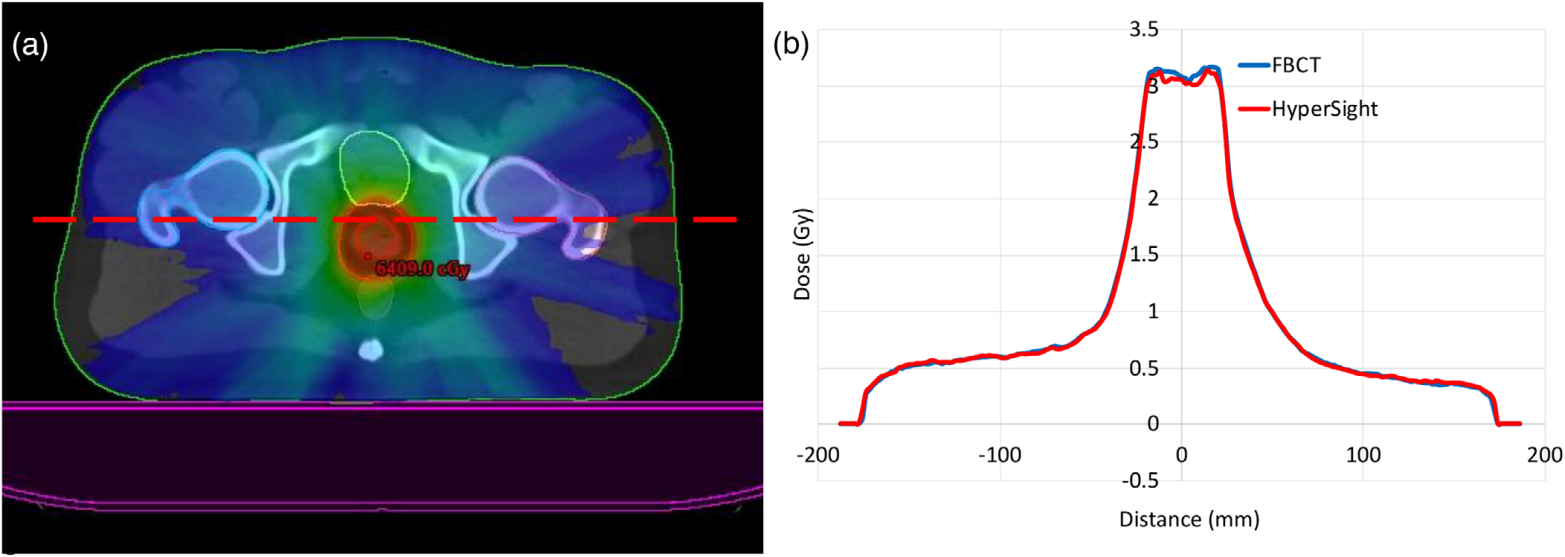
Dose profiles through the same plan calculated on HyperSight and fan beam CT (FBCT) images. (a) shows the dose distribution on the FBCT with the location of the dose profile shown at the red dotted line. (b) Shows the dose profiles as measured using PTW Verisoft with the red profile for HyperSight and the blue profile for FBCT. The gamma was measured in PTW Verisoft for 3%/3 mm, 2%/2 mm, and 1%/1 mm with passing rates of 100%, 99.0%, and 94.2% respectively.
